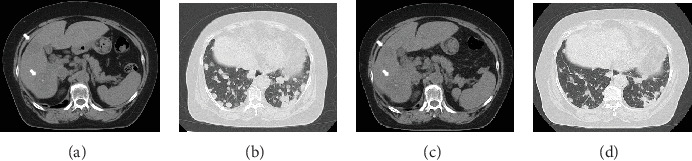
Abdominal and chest CT before the administration of panitumumab (a, b). Stable disease after therapy (c, d). Pulmonary metastasis was slightly reduced after panitumumab treatment. Arrows: lesions in the liver.
